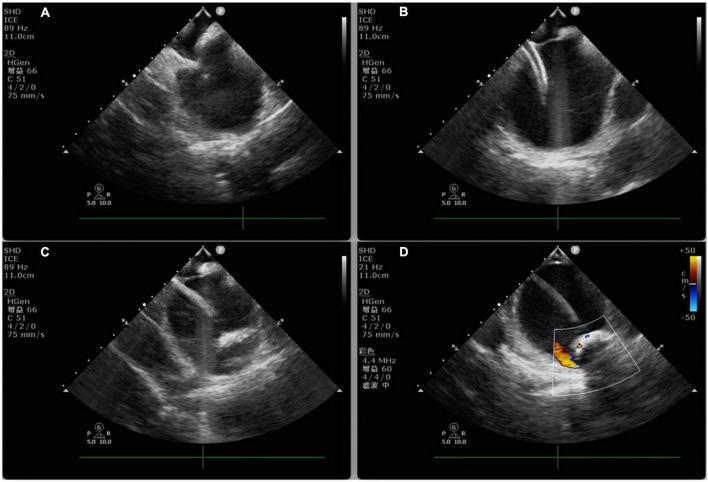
Intracardiac echocardiography-guided cryoablation. (A) ICE-guided low transseptal puncture; (B) cryoablation sheath delivered through the low transseptum; (C) cryoballoon delivered into the left superior pulmonary vein under ICE guidance; (D) ICE color Doppler ultrasound applied to assess cryoballoon closure.
